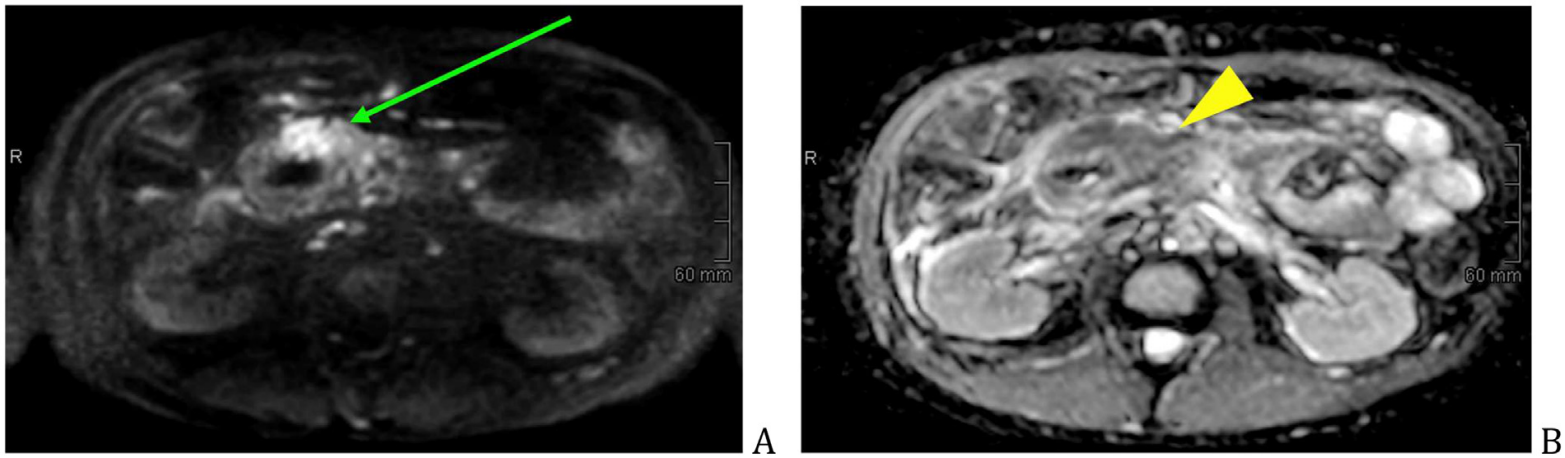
Pancreatic head mass restricted on DWI (green arrow) (A), with low-values of ADC (yellow arrowhead) (B).
